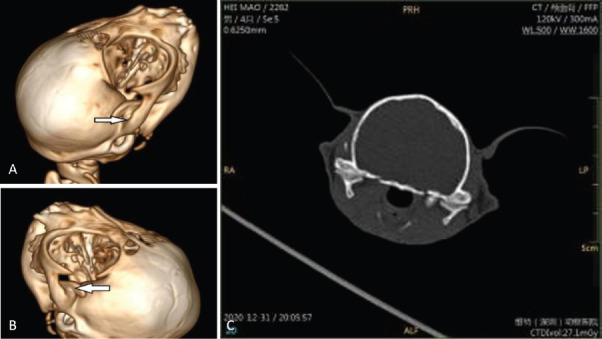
Figure 1. (A) 3D CT reconstruction, image on the right, arrows showing the malformed fusion of the right mandibular coronal process and zygomatic arch. (B) 3D CT reconstruction, left image, arrows show the malformed fusion of the left mandibular coronal process and zygomatic arch. (C) CT 2D sectional view.
